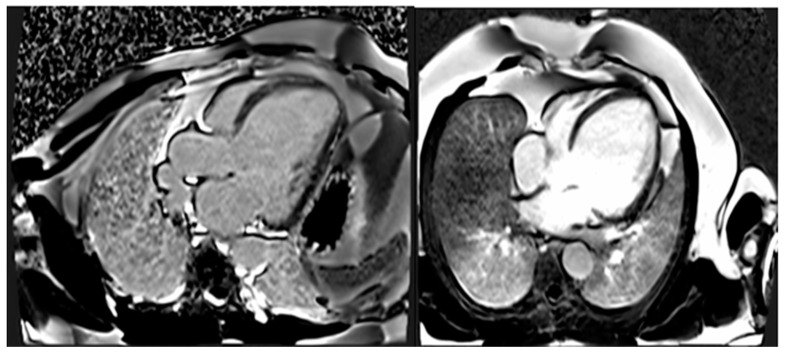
Cardiac magnetic resonance images, 4-chamber (horizontal long axis) and 2-chamber (vertical long axis). Dilated left ventricle with hyper-trabeculations of the antero-lateral wall; late gadolinium enhancement shows septal mid-wall fibrosis, suggestive of idiopathic dilative cardiomyopathy with left ventricular non-compaction.
